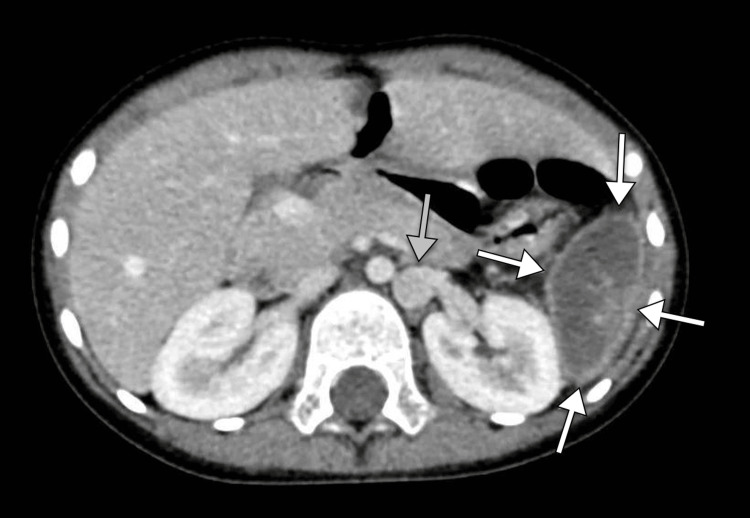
Axial CT image of the abdomen displaying a homogeneous hypodense mass with an enhancing wall (white arrows) and a left-sided inferior vena cava (gray arrows). CT, computed tomography.
